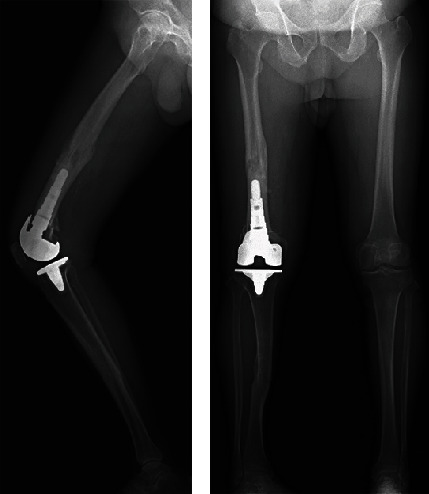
Postoperative radiograph. (a) Lateral view: Femoral and tibial implants are implanted at 0.5° varus. (b) Anteroposterior view: Hip–knee–ankle angle is 1° varus, the femoral implant is implanted at 0.5° flexion, and the tibial implant is implanted at 0° posterior slope.
